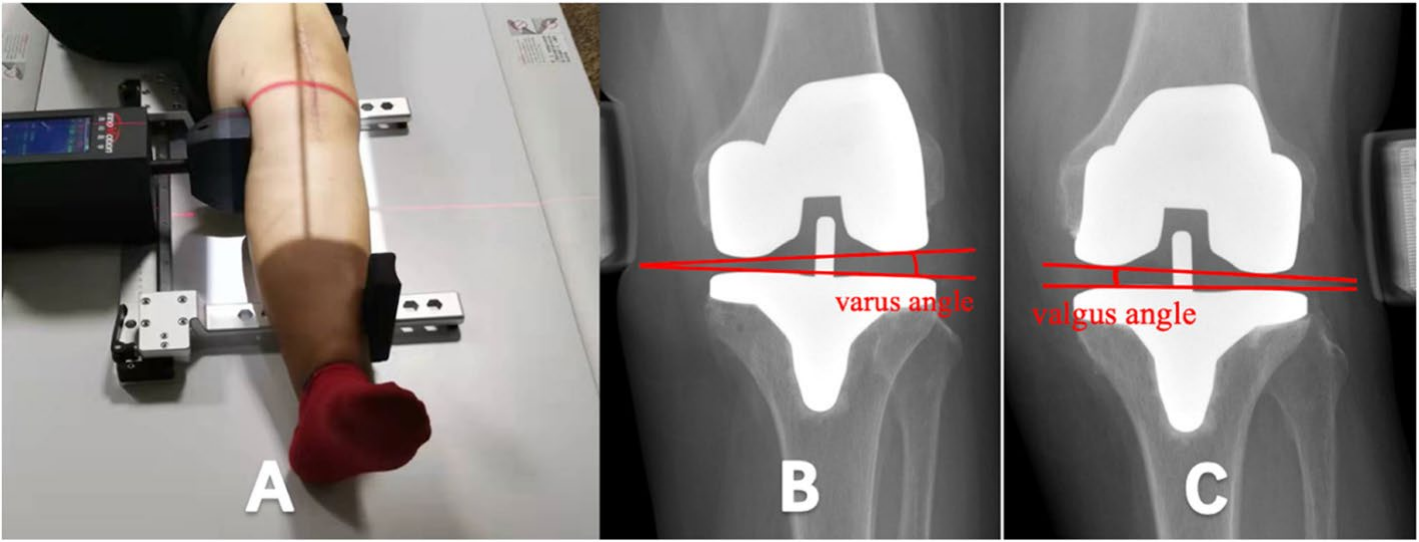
A: X-ray of stress position taken when 100N force is applied with knee joint ligament measuring device. B: The measurement of the varus angle after TKA is the included angle formed by the line from the lateral femoral condyle to the medial femoral condyle and the parallel line of the tibial plateau. C: The measurement of the valgus angle after TKA is the included angle formed by the line from the medial condyle of the femur to the lateral condyle and the parallel line of the tibial plateau
